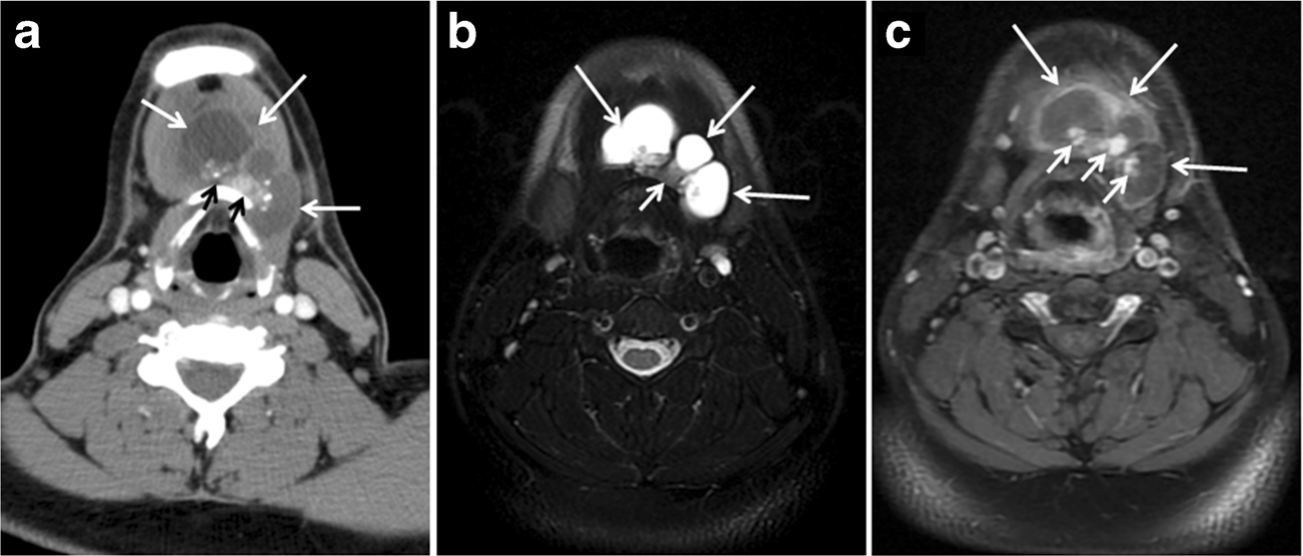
Papillary thyroid carcinoma arising from a thyroglossal duct cyst in a 28-year-old male. a Axial enhanced CT scan shows a large complex cystic lesion (*white arrows*) adherent to the anterior aspect of the hyoid bone. It has an enhancing mural solid nodules and calcifications (*black arrows*). There is no cervical lymphadenopathy. b Axial short tau inversion recovery (STIR) MRI image near the same level shows complex lesion of high signal intensity (*long white arrows*) with solid mural nodules (*short white arrow*). c Axial fat saturated T1 MRI image post contrast administration shows the complex cystic lesion with thick enhancing wall (*long white arrows*) and enhancing mural nodules (*short white arrows*)
